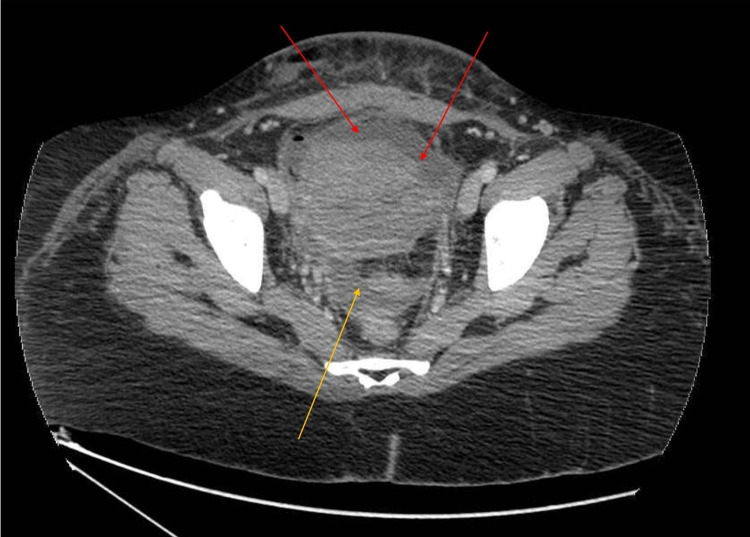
CT abdomen revealing pre-uterine (red arrows) and pelvic collections (yellow arrows)
